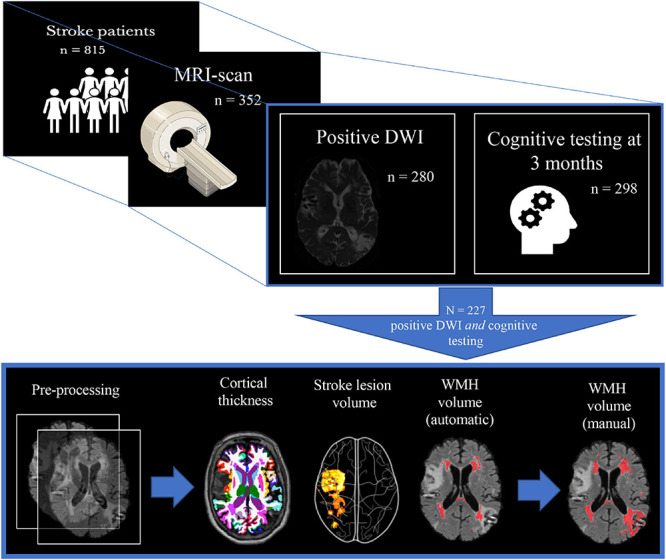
Study population selection with subsequent imaging processing. The figure depicts the inclusion process and subsequent imaging processing of the final study sample. From 815 participants, 352 underwent a study-specific MRI-scan, where 280 of these also had the stroke lesion visible on DWI, and 298 had cognitive testing at 3 months. This led to a final study population of 227 whereupon the following steps involved pre-processing of T1 + FLAIR MRI images: cortical thickness measures (FreeSurfer), stroke lesion volume (IKT SNAP), WMH volume (FSL BIANCA), and finally manual editing of the FSL BIANCA output. WMH, white matter hyperintensities.
